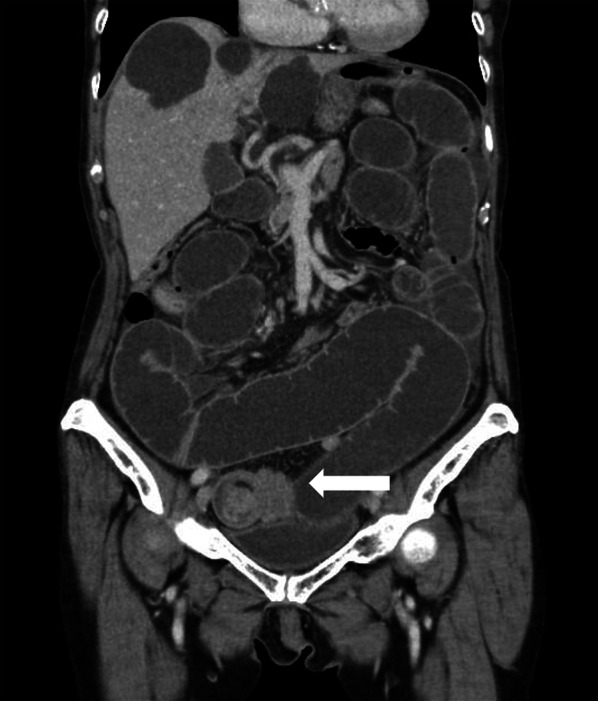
Late-phase contrast-enhanced CT. Target sign is detected in the small intestine in right lower abdomen (arrow), which indicates intussusception. The small intestine is diffusely dilated. No ascites or intestinal ischemic changes are seen
